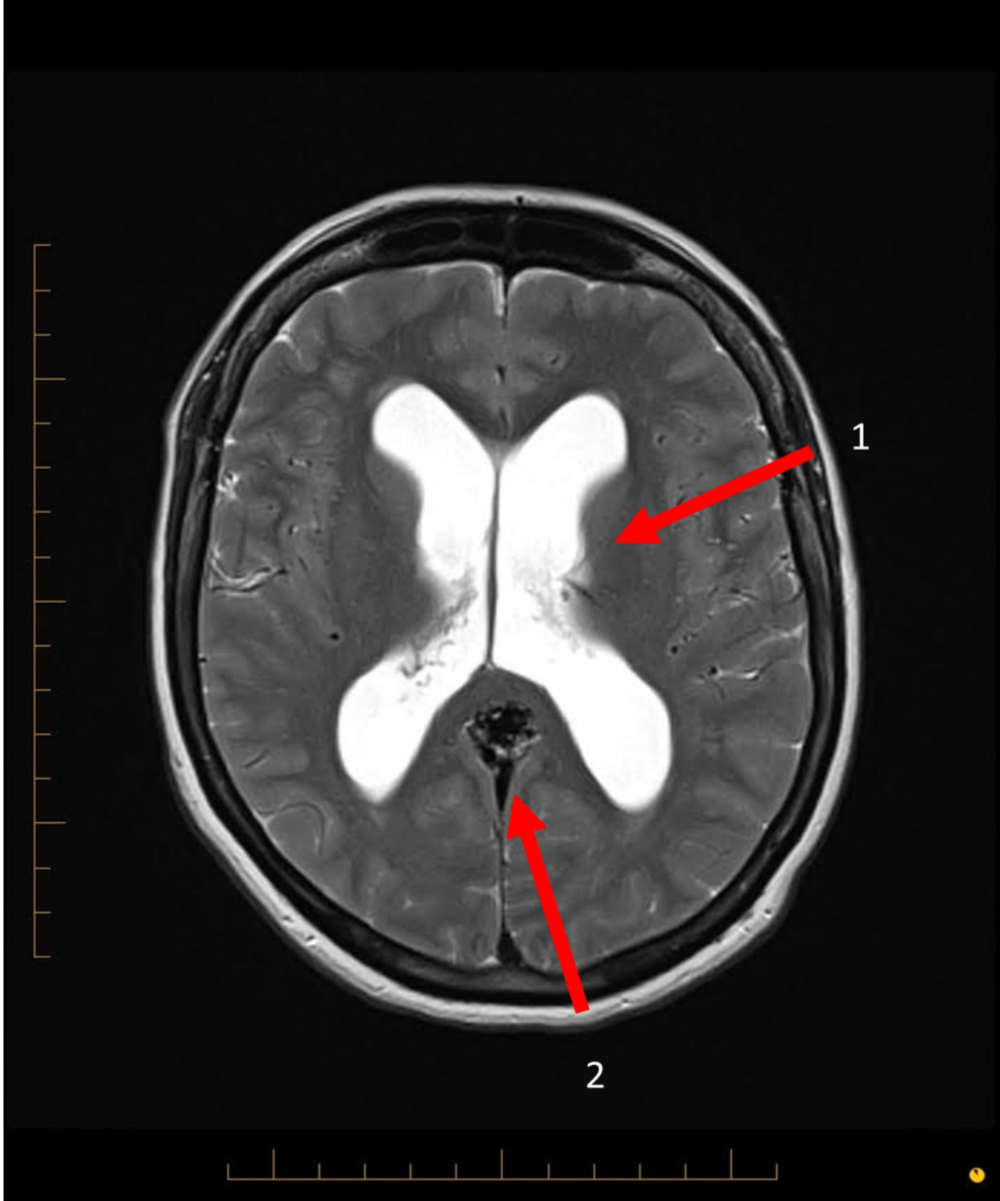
Magnetic resonance imaging axial view of the brain. Arrow 1 demonstrates the dilated hydrocephalic ventricles. Arrow 2 demonstrates the arteriovenous malformation.
